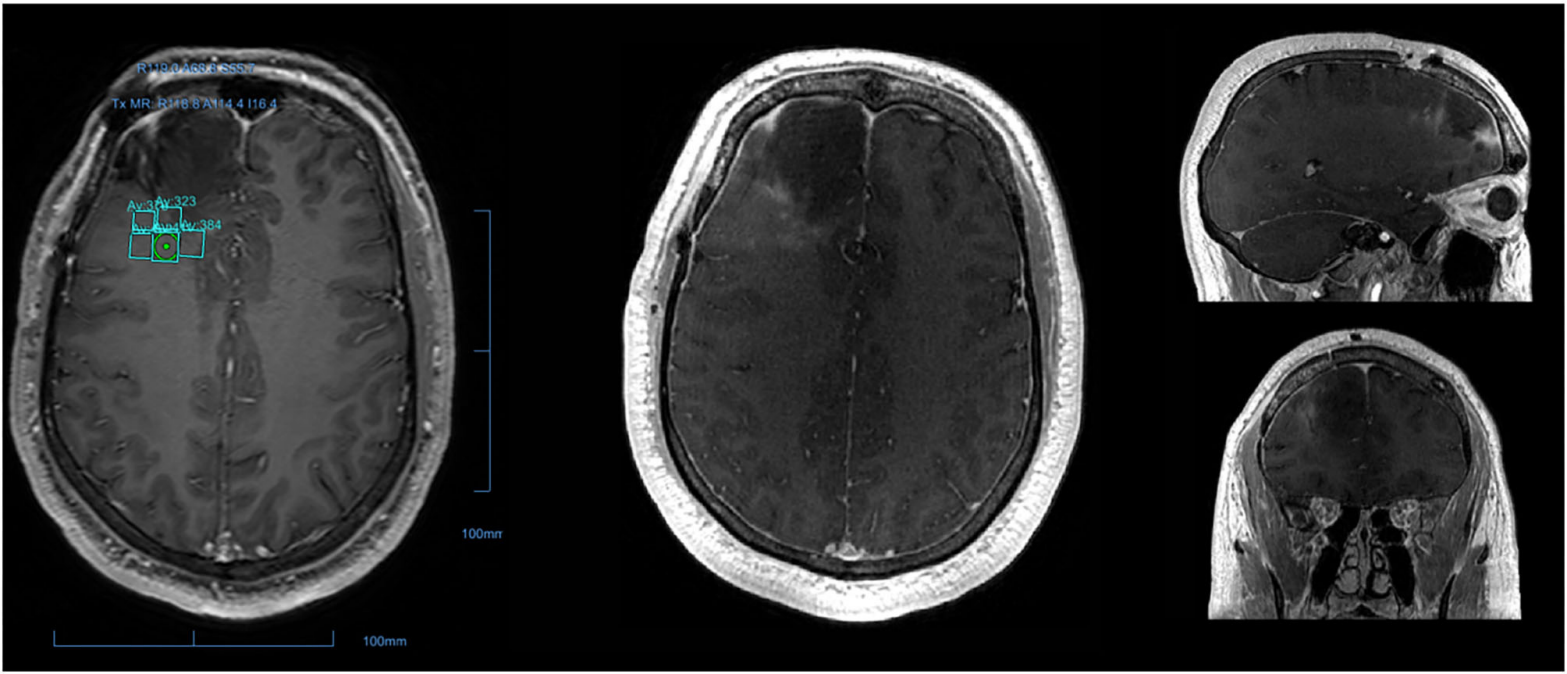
Post-operative peritumoral BBB disruption via MR-guided focused ultrasound. T1-weighted MRI from a patient who underwent surgery for glioblastoma followed by sonication of the resection boundary. The sonication field encompassed the 1 × 1 cm grid demonstrated, with nine sonication spots in each. Gadolinium, administered immediately after sonication was complete, was used to confirm precise disruption of the blood-brain barrier as indicated by the nine small areas of gadolinium leakage.
